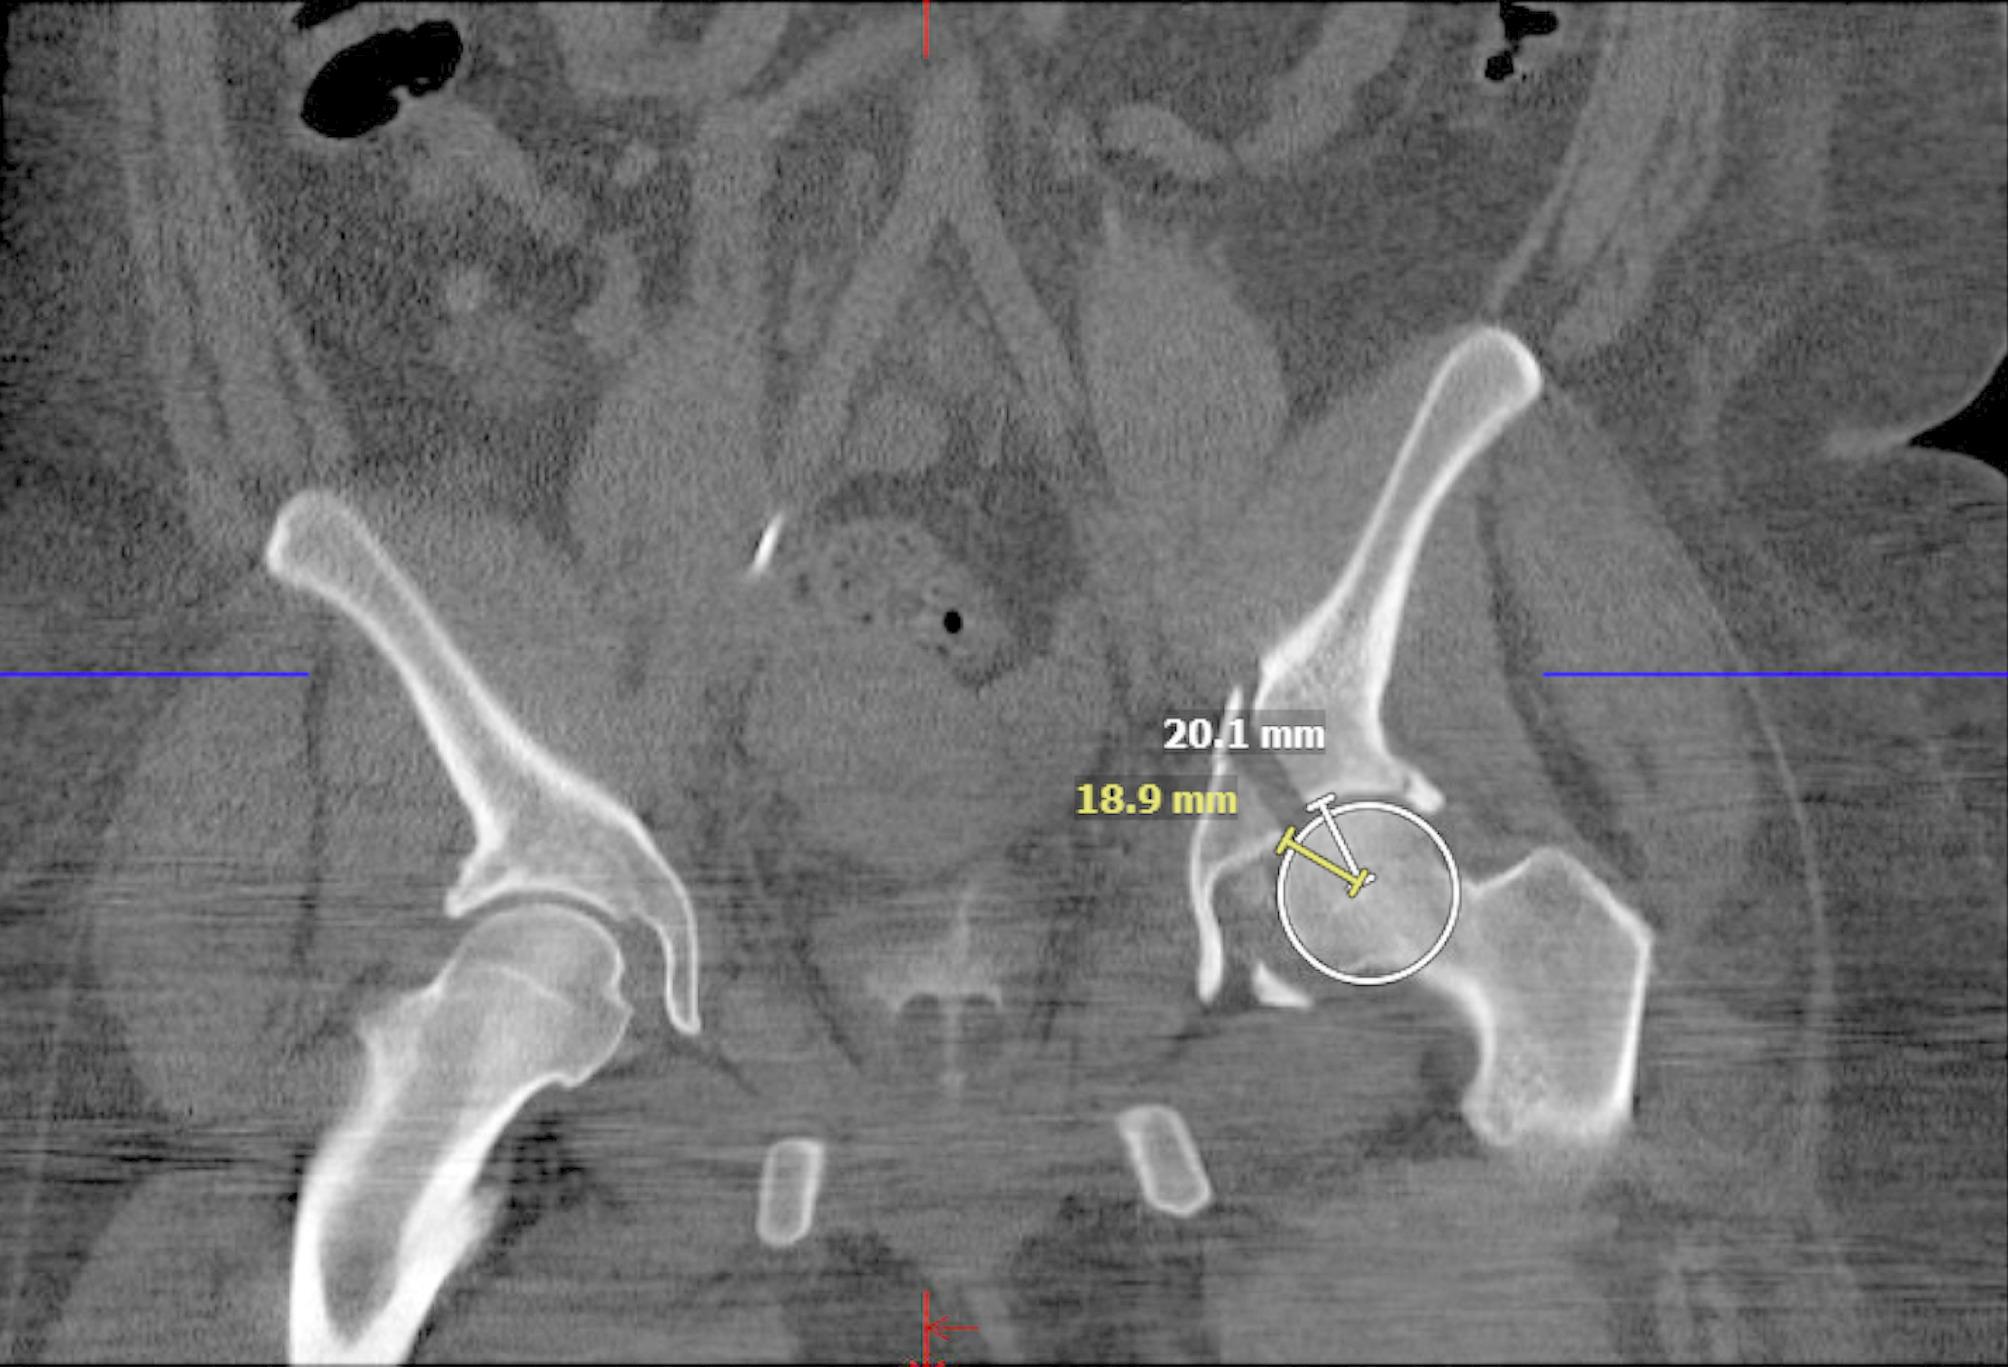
Step-off measurement in coronal computed tomography axis
